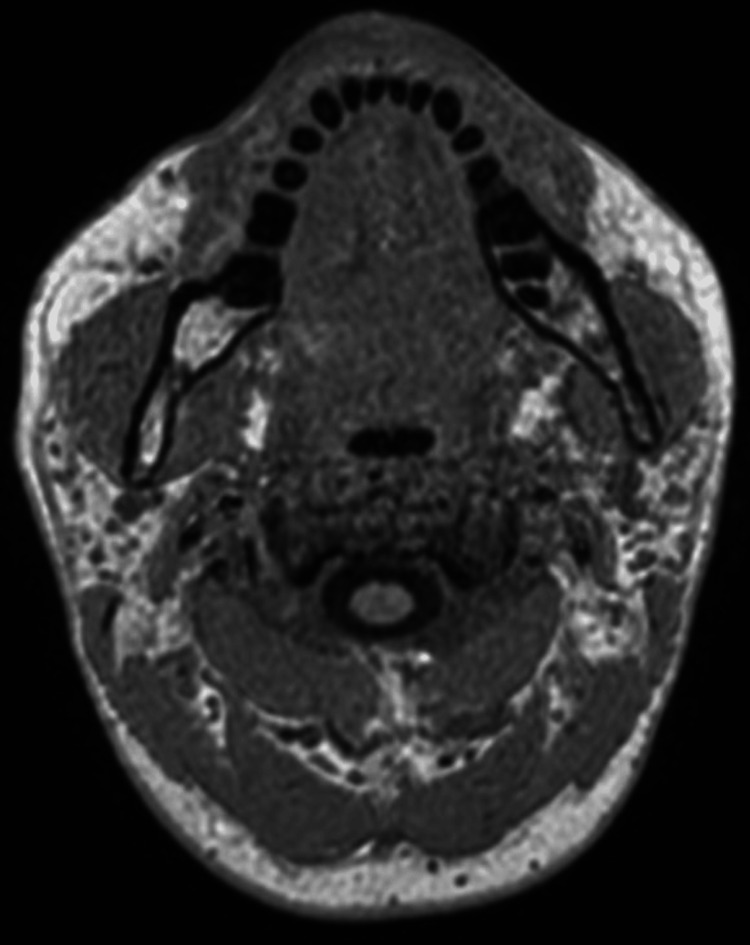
T1-weighted image depicts a large area of fat signal, with almost no normal gland. Staging system for conventional imaging of the parotid glands proposed by Rao et al. [14], grade 4.
